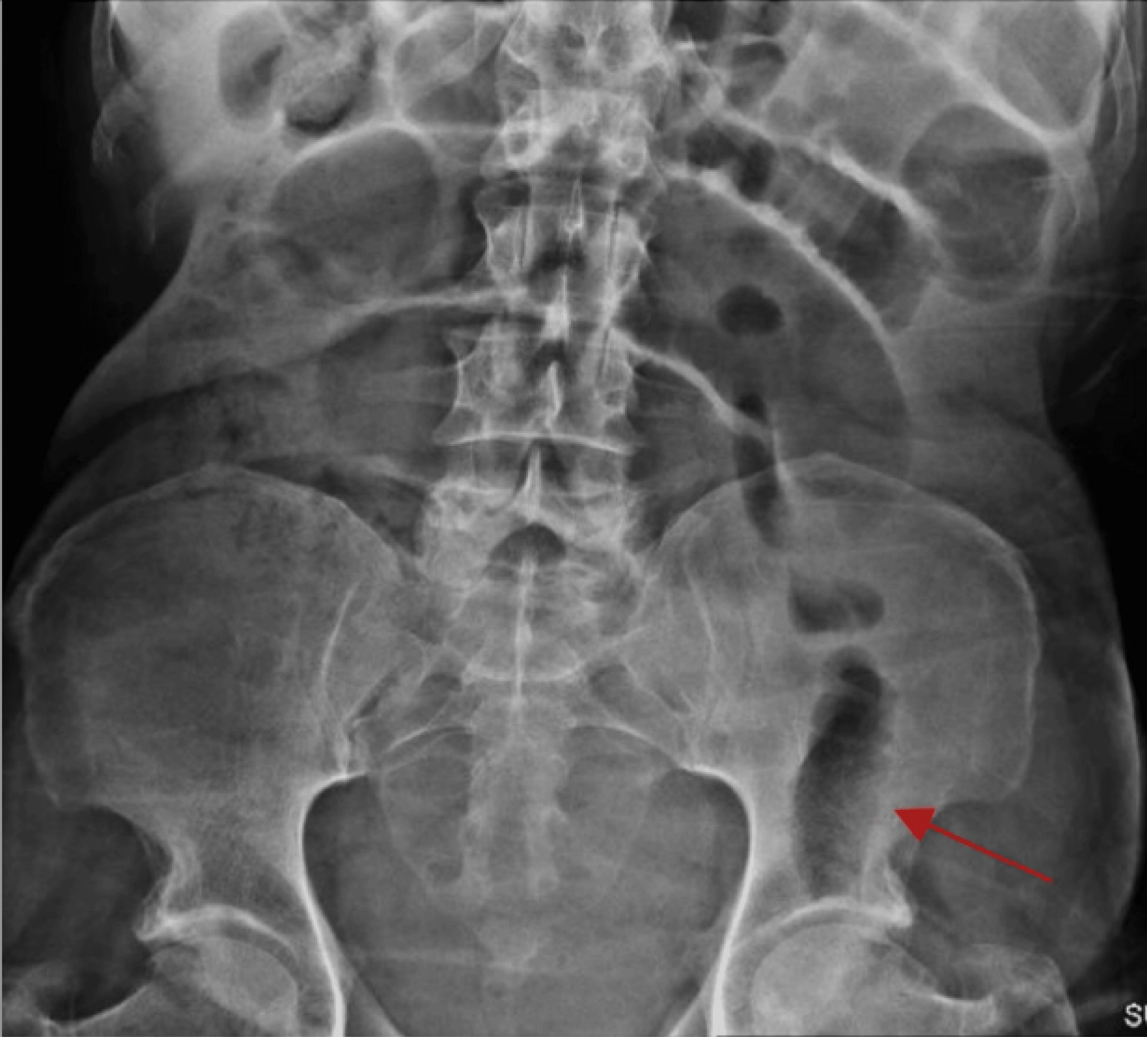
Abdominal X-ray showing small bowel on the left lower quadrant (red arrow)
